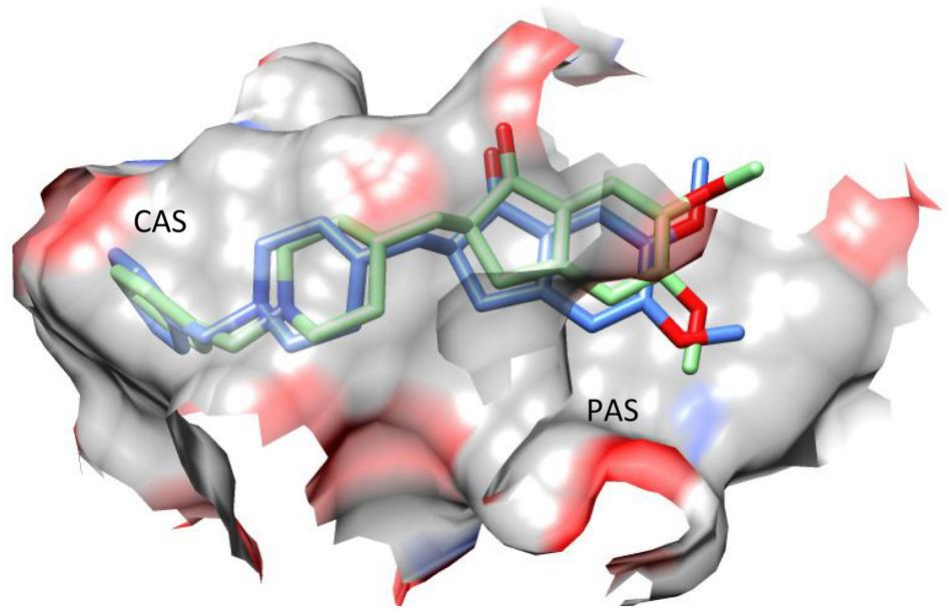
Superposition of the X-ray (blue) and docked (light green) structures of donepezil within the active site of AChE.
